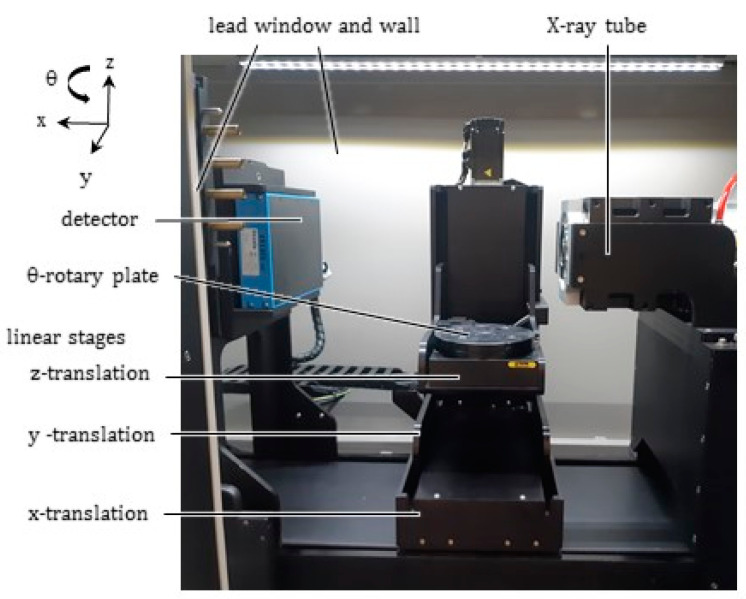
Inner view of the cabinet for X-ray microtomography available at Politecnico di Milano, showing the 4-dof motorized system for the sample positioning (see the reference frame), the tube and the movable detector.
